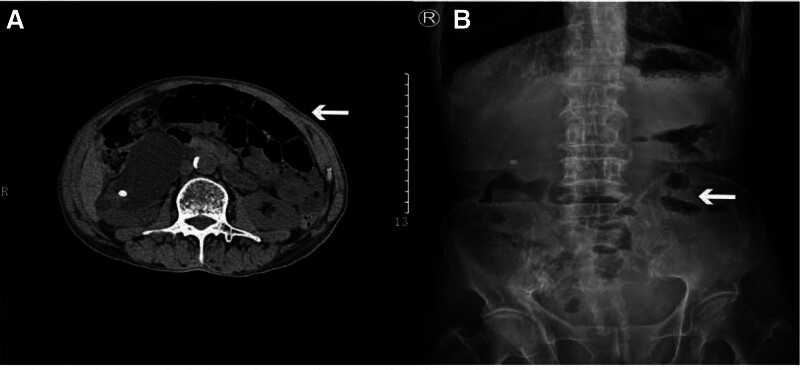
The imageological examination of case 1 patient. (A) Abdominal CT (taken on December 3, 2023) and (B) abdominal x-rays (taken on December 11, 2023) suggesting “intestinal obstruction.” CT = computed tomography.
